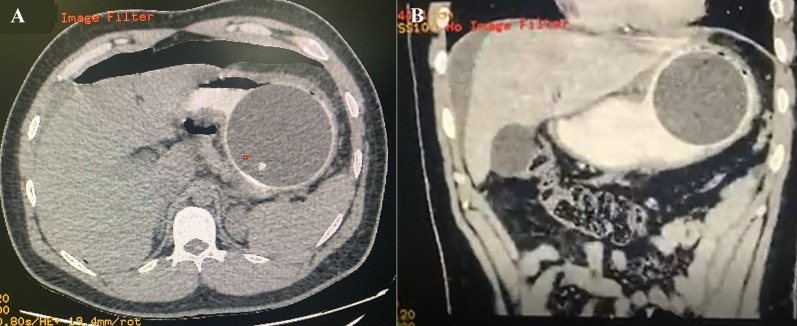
A CT, Free air in the abdomen. B CT, Perihepatic liquid along with the intragastric balloon
